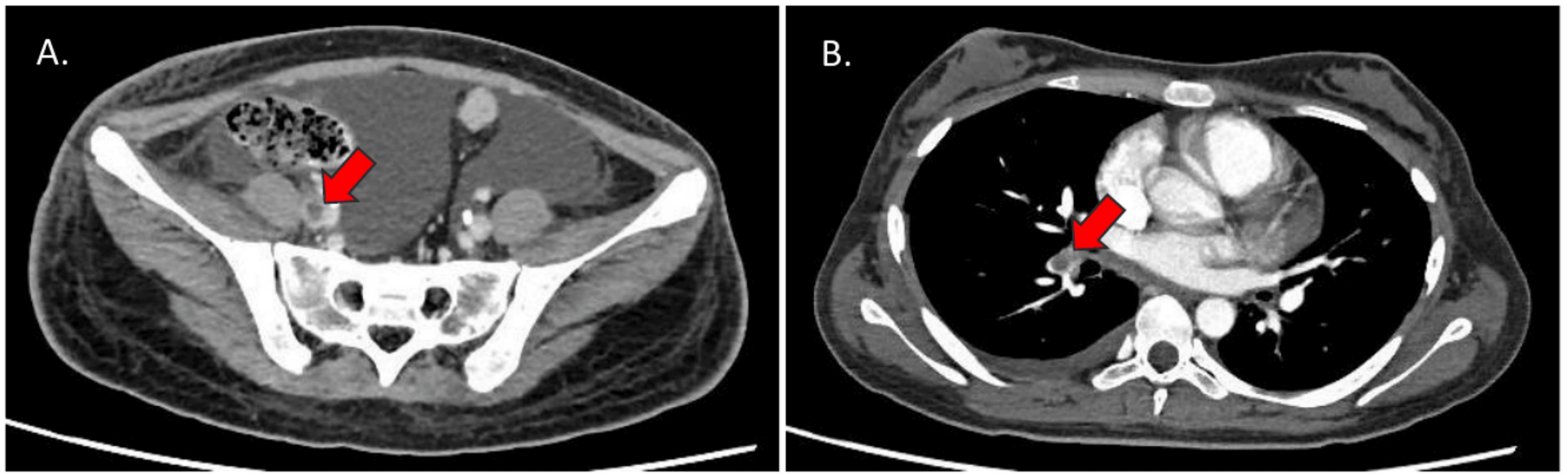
Contrast-enhanced CT findings of iliac vein thrombosis and pulmonary embolism. (A) Contrast-enhanced CT of the pelvis demonstrates thrombi in the right common iliac vein (arrows). (B) Contrast-enhanced CT of the chest reveals filling defects in the pulmonary arteries, consistent with pulmonary embolism (arrows).
